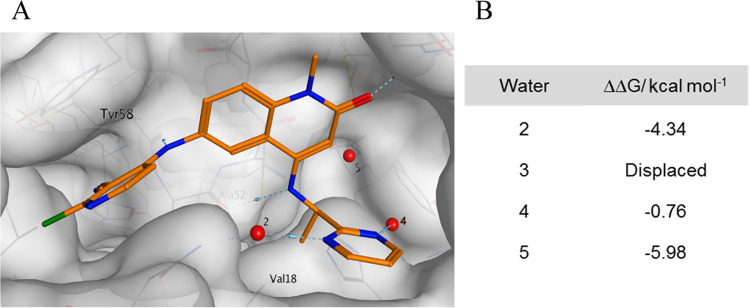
(A) X-ray structure of 13e (PDB: 7OKL, resolution 1.25
